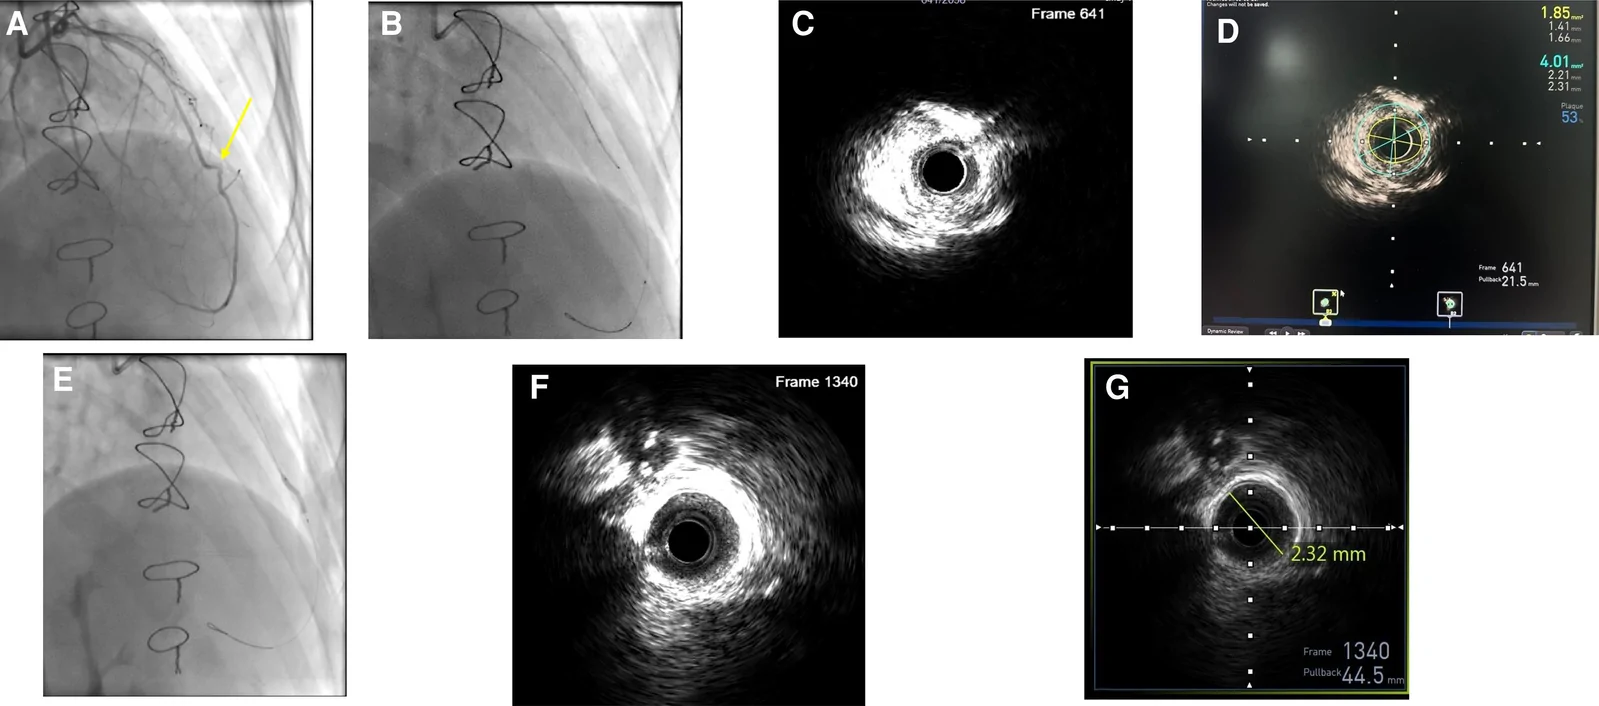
Pre-percutaneous coronary intervention coronary angiography and intravascular ultrasound assessment of the left anterior descending artery lesion. *(A)* Right anterior oblique cranial view of coronary angiography showing the mid-left anterior descending artery lesion (arrow). *(B)* Insertion of the intravascular ultrasound (Opticross) 3F probe into the target lesion of the left anterior descending artery. *(C)* Intravascular ultrasound imaging reveals the most severe stenosis, with a concentric, fibrotic appearance. *(D)* Intravascular ultrasound measurement of the minimum lumen area, 1.85 mm^2^, without an atherosclerotic phenotype. *(E)* Pre-dilation of the left anterior descending artery lesion was performed using a semi-compliant Shun Loach PTCA balloon, 2.0 × 18 mm (Shunmei Medical Co., China). *(F, G)* Immediately after pre-dilation, intravascular ultrasound showed a vessel diameter of 2.32 mm at the treated segment.
